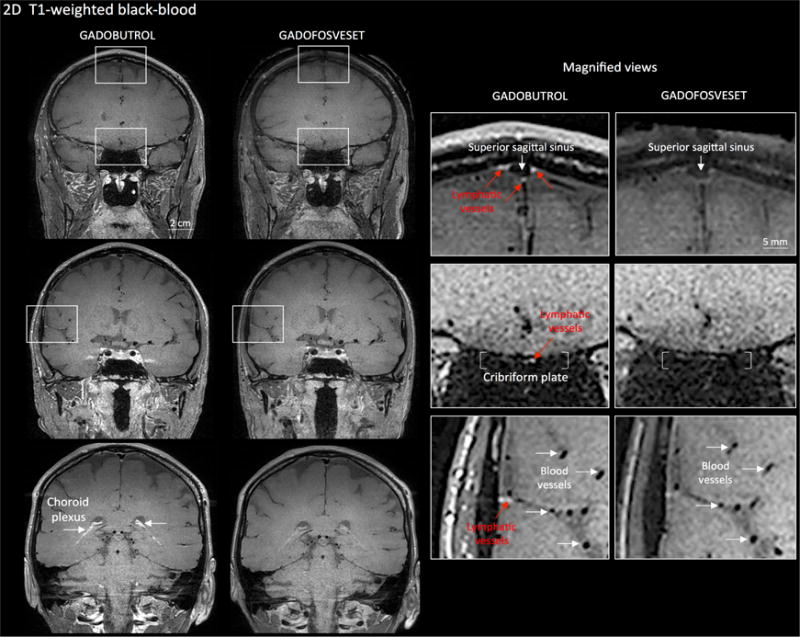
Gadobutrol vs. gadofosveset in MRI visualization of dural lymphatic
